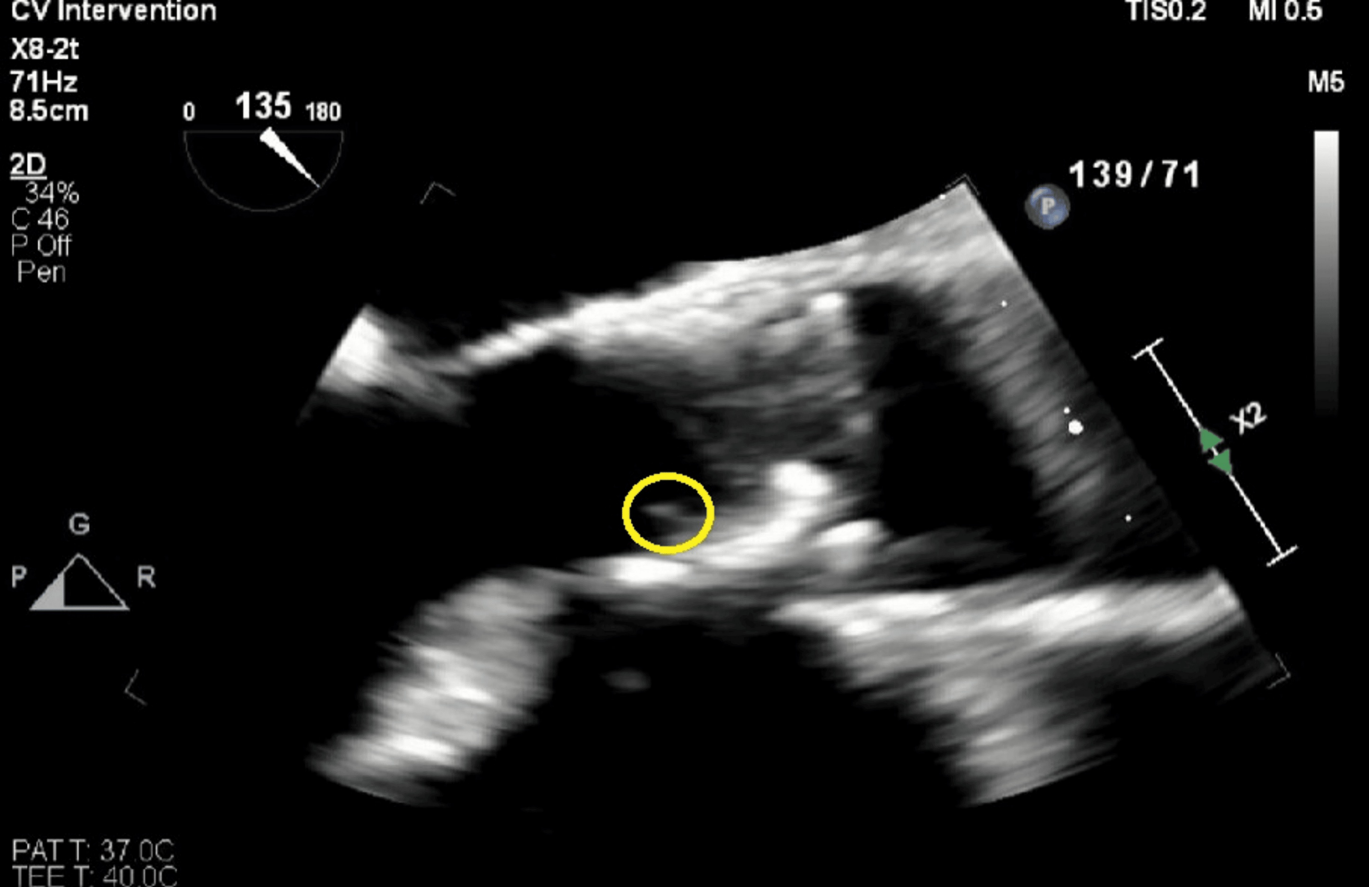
Another transesophageal echocardiogram (TEE) imaging confirming the presence and location of vegetation on the prosthetic aortic valve (yellow circle).
